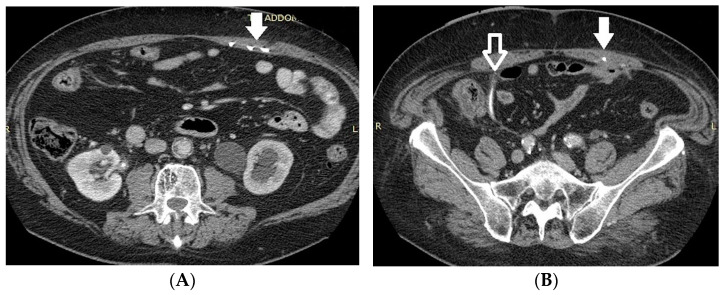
Bilateral splint in a patient with a history of radical cystectomy and ureterocutaneostomy. Axial CT scans. On the left side (A), the splint is seen in the abdominal wall (solid arrow), while on the right side (B), the splint is seen in place (empty arrow). Left-sided hydronephrosis persists.
